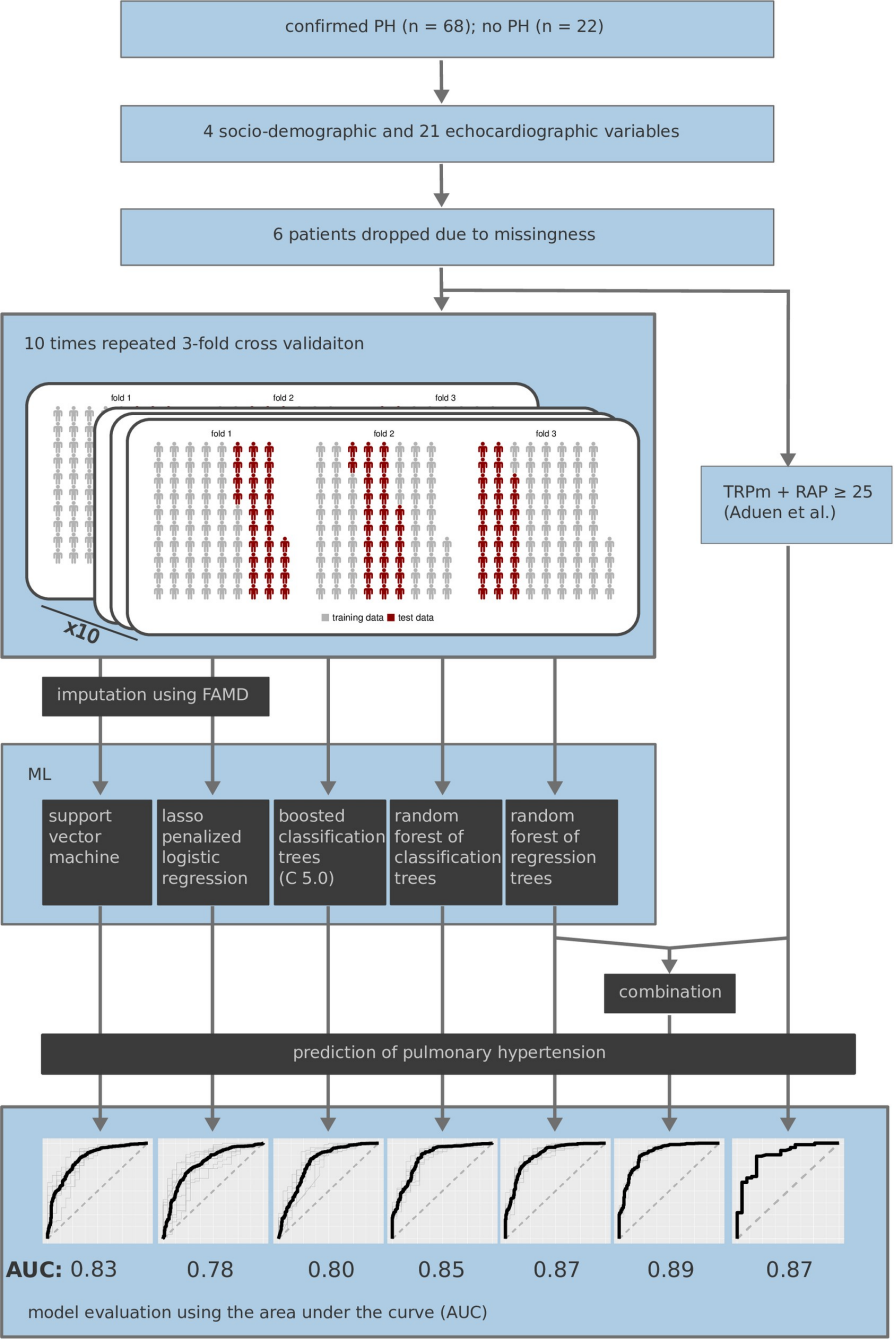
Overview procedure and main results. The data set comprises measurements of 68 patients with confirmed PH and 22 patients without PH. Four socio-demographic and 21 echocardiographic variables were measured. Six patients were dropped due to the high degree of missingness. As reference the formula by Aduen et al. was evaluated. Five ML methods were applied and evaluated using a 10 times repeated 3-fold CV scheme. Two ML methods required an imputation as pre-processing step within each fold of the CV. The predictions of the random forest of regression trees have additionally been combined with the predictions by Aduen et al.
